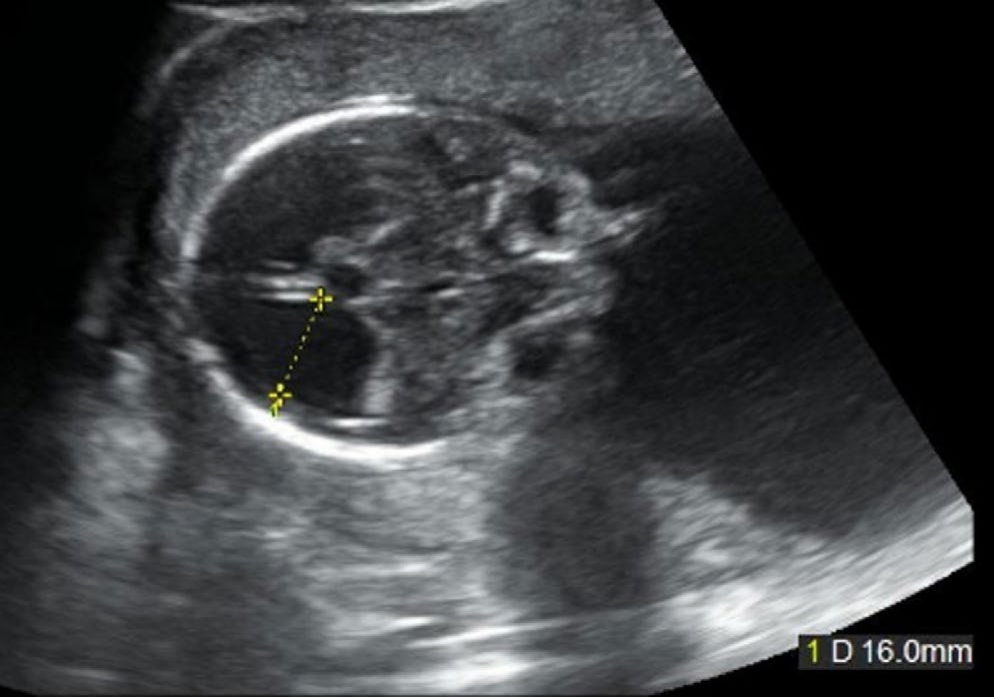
Ultrasonography at 20 weeks of gestation revealed an enlargement of the posterior horn of the lateral ventricle to 16 mm.
